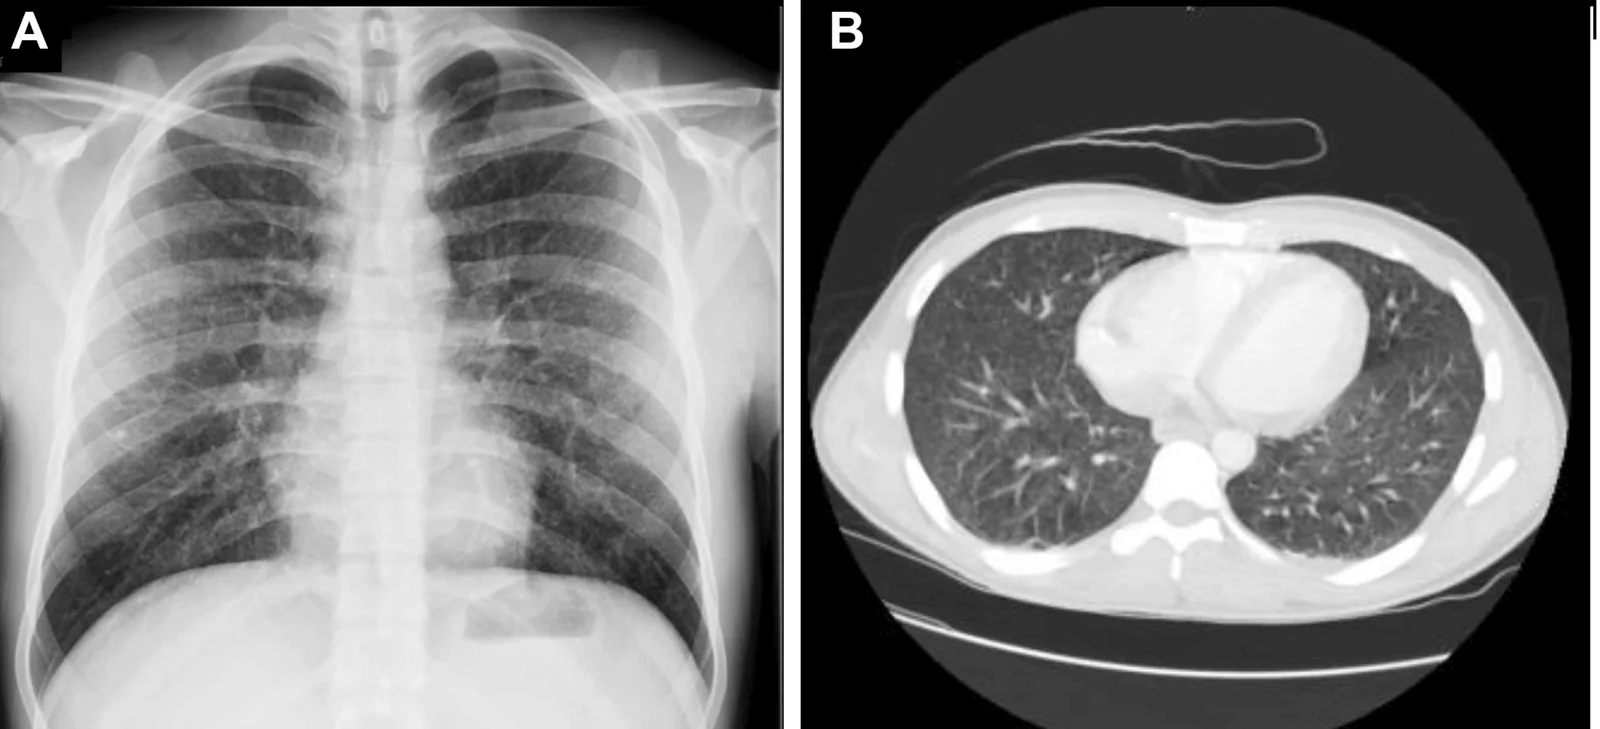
(A) PA chest X-ray and (B) CT imaging of the chest at the time of clinical presentation (A) PA chest X-ray at the time of the current presentation. (B) CT of the chest. Both images show diffuse, bilateral miliary distribution of micronodules in the lungs, suggesting miliary tuberculosis or other atypical infections such as histoplasmosis. PA: posterior-anterior; CT: computed tomography
